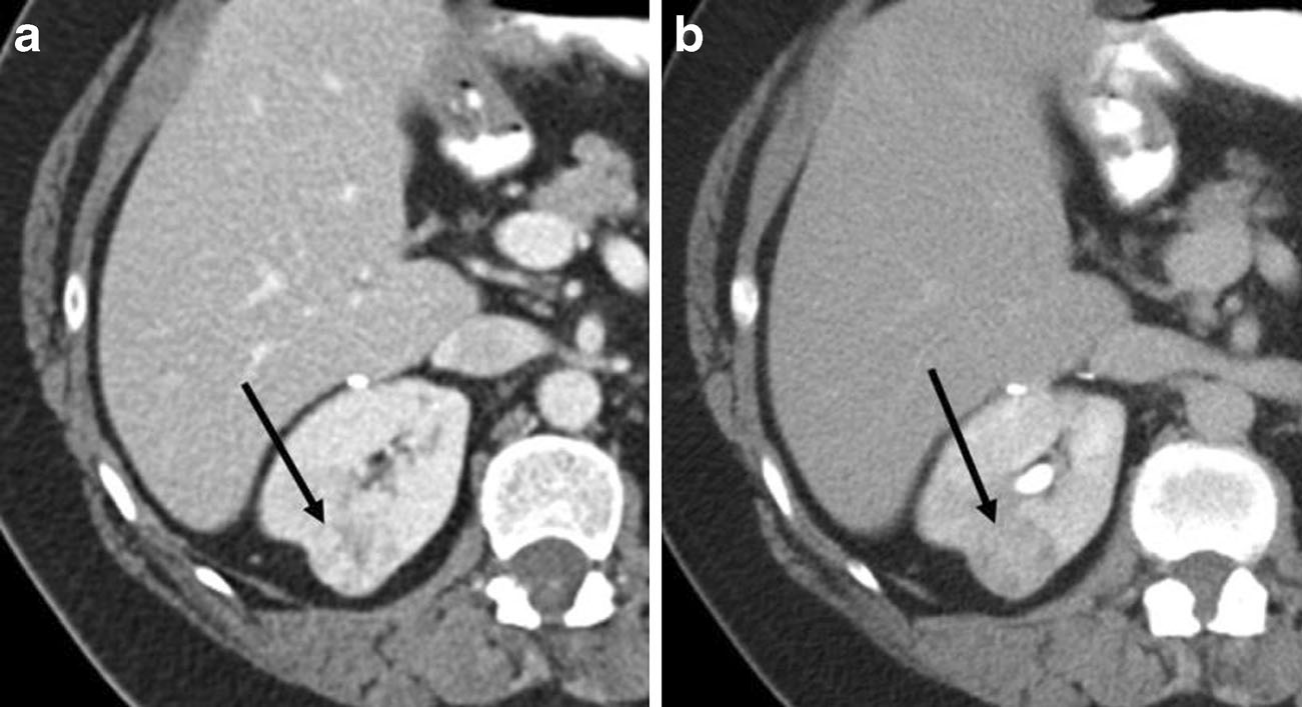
A 38-year-old woman with clear cell RCC of the right kidney mimicking segmental enhancement inversion (*see* Fig. 2). a The nephrographic phase of the axial CE-CT demonstrates avid contrast enhancement and an area of central low attenuation (*arrow*). b The enhancing area in the nephrographic phase (a) washes out to low attenuation in the delayed phase. The low attenuation area in the nephrographic phase shows relatively high attenuation (*arrow*)
